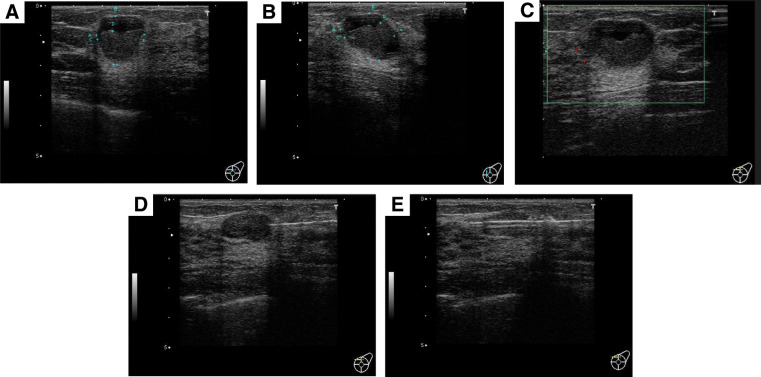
Breast US (A–E). US revealed a cystic mass measuring 16.8 × 21.4 × 16.1 mm and a solid mass measuring 16.3 × 17.4 × 12.2 mm in the upper-inner quadrant of the left breast. Color Doppler US showed a peripheral vascular enlargement and a lack of central vascularization. The core needle was inserted parallel to the chest wall and was fired as the needle tip reached the edge of the tumor so as to keep the tumor from shifting. US, ultrasonography
